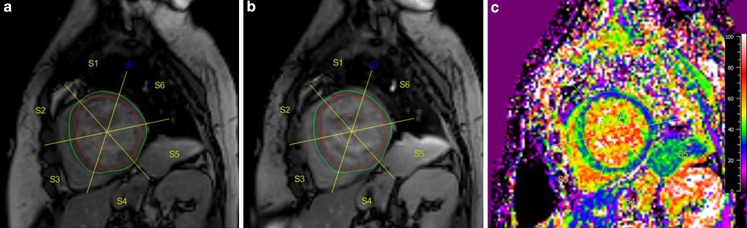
a–c Cardiac magnetic resonance imaging. Cardiac magnetic resonance imaging with T1 mapping in a female breast cancer survivor, treated with anthracyclines. Extracellular volume fraction (ECV) is a non-invasive measurement of diffuse myocardial fibrosis and can be calculated from the haematocrit; pre-contrast (a), post-contrast (b) T1 maps. In this patient, the ECV map (c) reveals diffuse elevated ECV values up to 42% (normal is <28%), in particular in the septal segments, reflecting widespread myocardial fibrosis after anthracycline exposure
